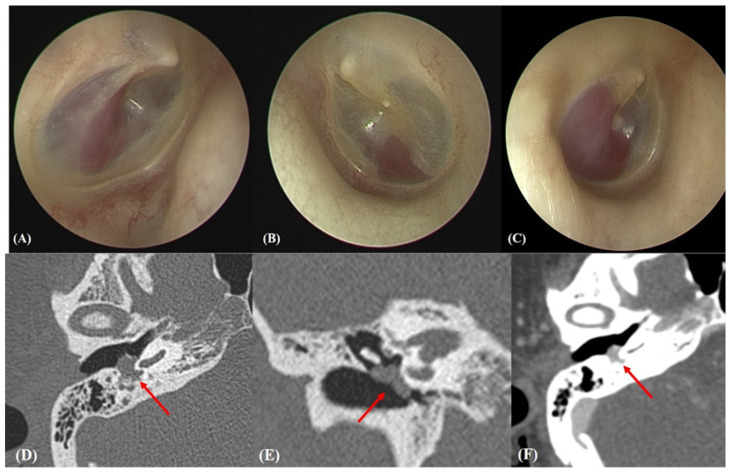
(A–C) Preoperative endoscopic views of subjects 4, 5, and 6, respectively. In each case, a reddish round mass abuts the tympanic membrane. (D–F) Preoperative temporal bone computed tomography (TB-CT) images of subject #6. The initial axial (D) and coronal (E) TB-CT images (without contrast enhancement) reveal an 8 mm-sized mass (arrows) in the right middle ear lateral to the cochlear promontory that abuts the long process of the incus, stapes, and tympanic membrane. (F) The initial axial TB-CT image (with contrast enhancement) reveals a middle ear mass suggestive of a glomus tympanicum tumor (arrow).
